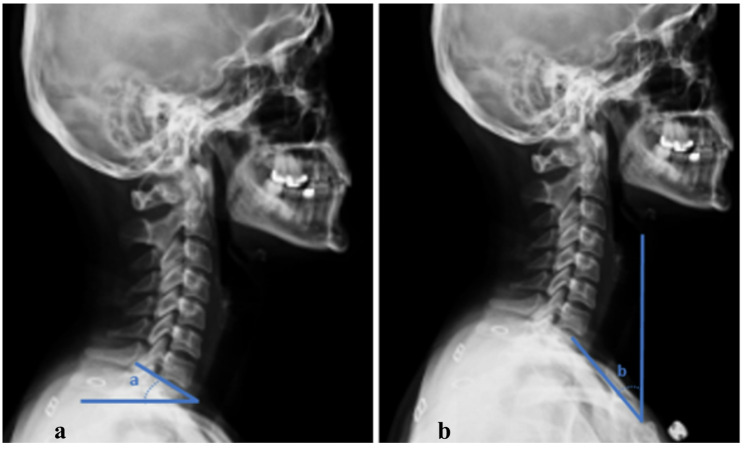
Measurement of T1 slope (a) and neck tilt (b) on a lateral cervical X-ray. T1 slope is defined as the angle between the superior endplate of the T1 vertebra and the horizontal plane on a standing lateral radiograph. T1 tilt is defined as the angle between a line connecting the midpoint of the superior endplate of T1 to the upper end of the sternum (manubrium) and the vertical axis
